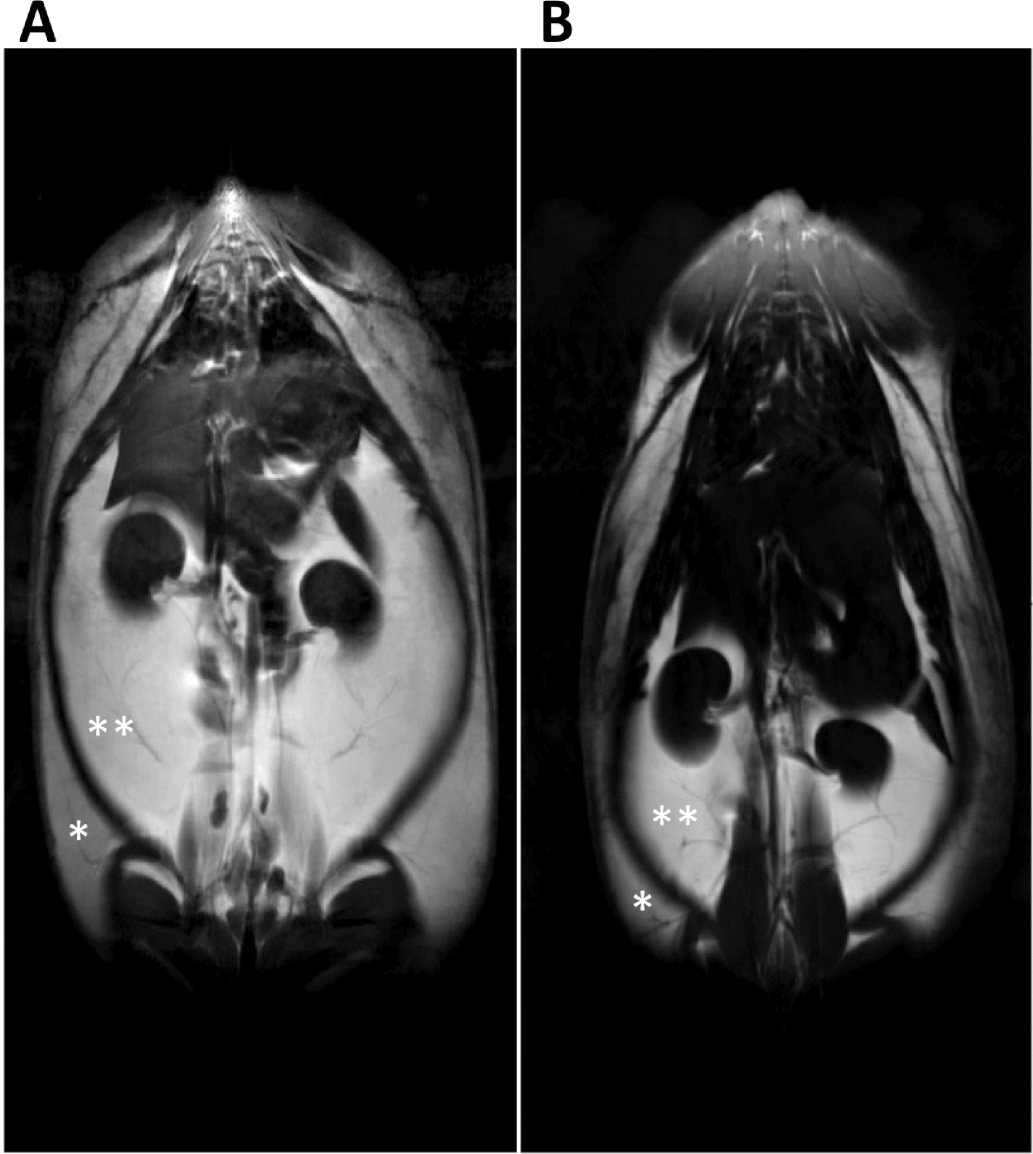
Water-suppressed MRI images at week 25 post-birth of fat distribution. In (A) male offspring from hypoxic dams fed the HFD and (B) male offspring from normoxic control dams fed the control chow diet. (peritoneal fat; * subcutaneous fat).
